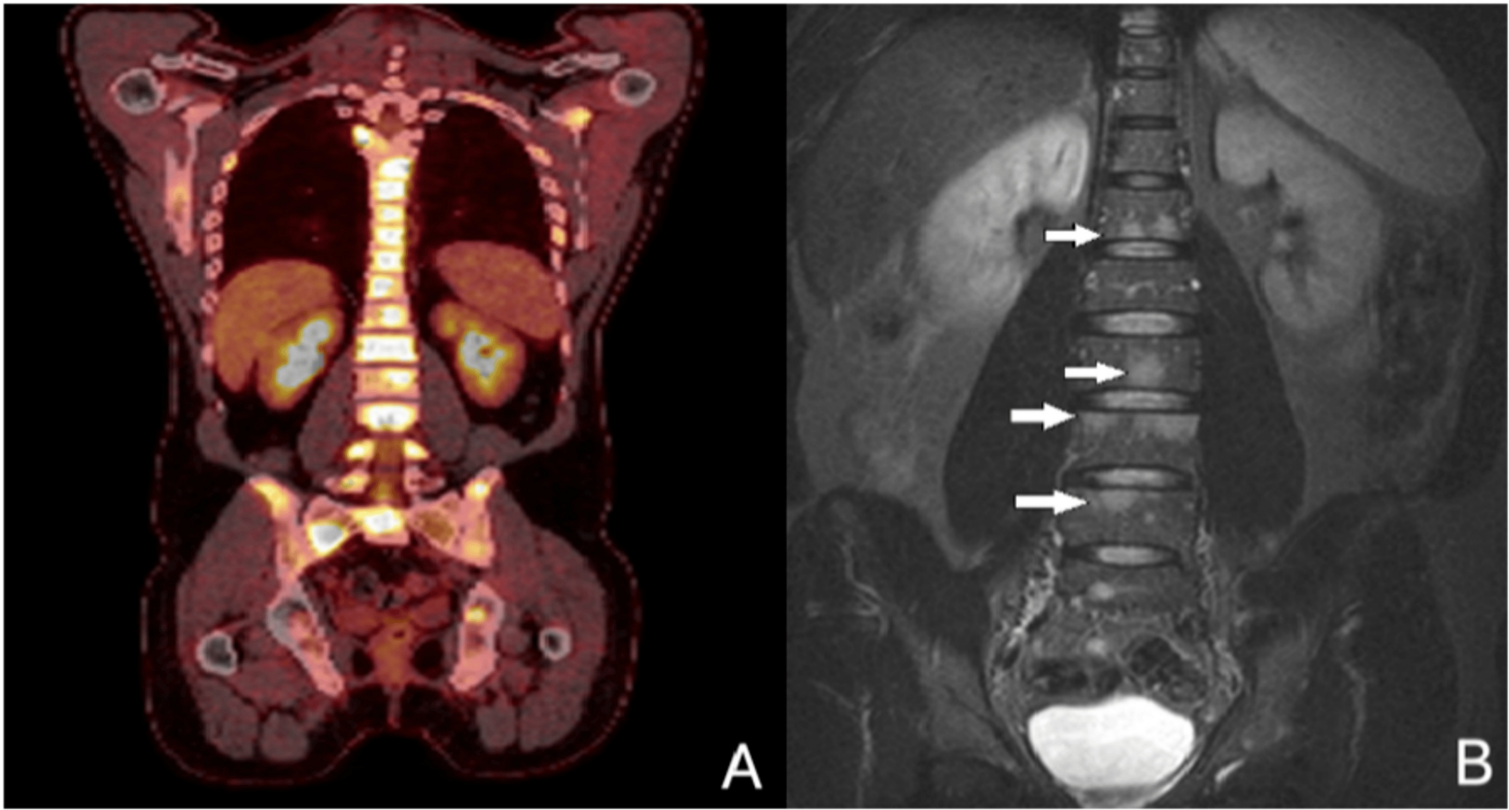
FDG-PET/CT scan. FDG-PET (Fluorodeoxyglucose Positron Emission Tomography) CT scan displaying multiple hypermetabolic bone lesions throughout the spine, in both scapulae and iliac bones (panel A). Coronal MRI scan of the spine demonstrating multiple metastatic bone-lytic lesions, exhibiting a high signal intensity in T2-weighed images (white arrows – panel B).
